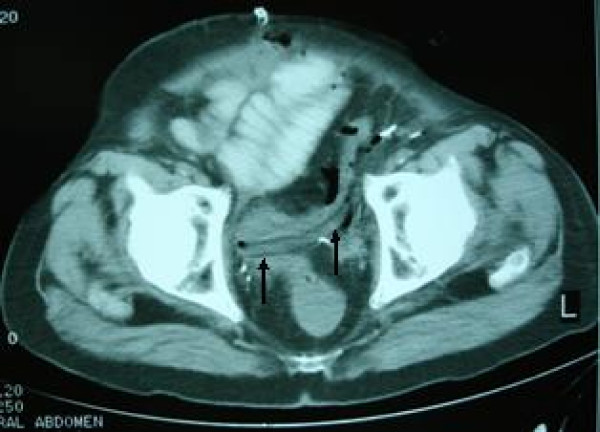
CT scan at case presentation. Computed tomography showing the drain abutting the sigmoid colon.
